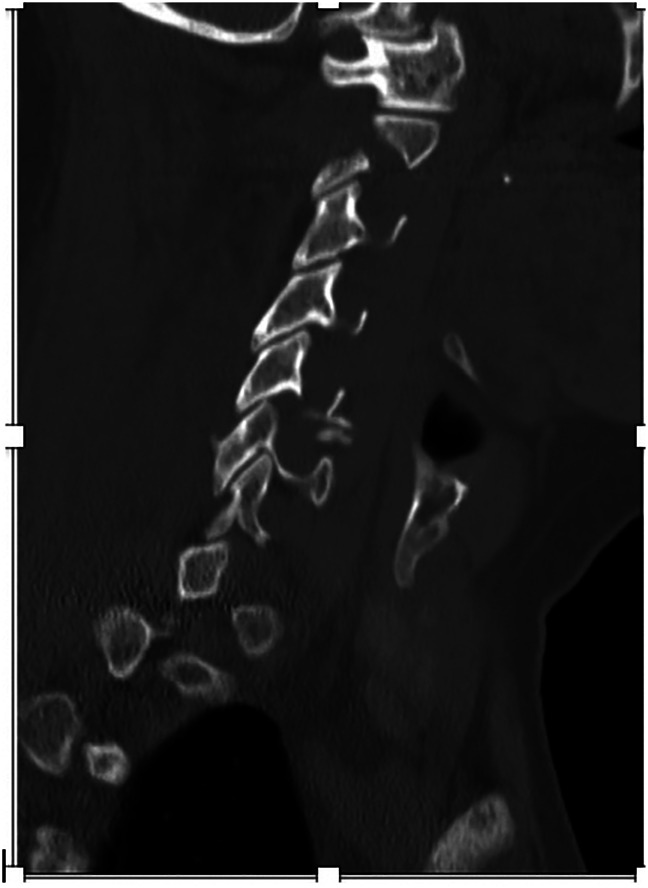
Exeter CT—sagittal CT scan, made with the patient supine, reported as showing widespread degenerative changes.
